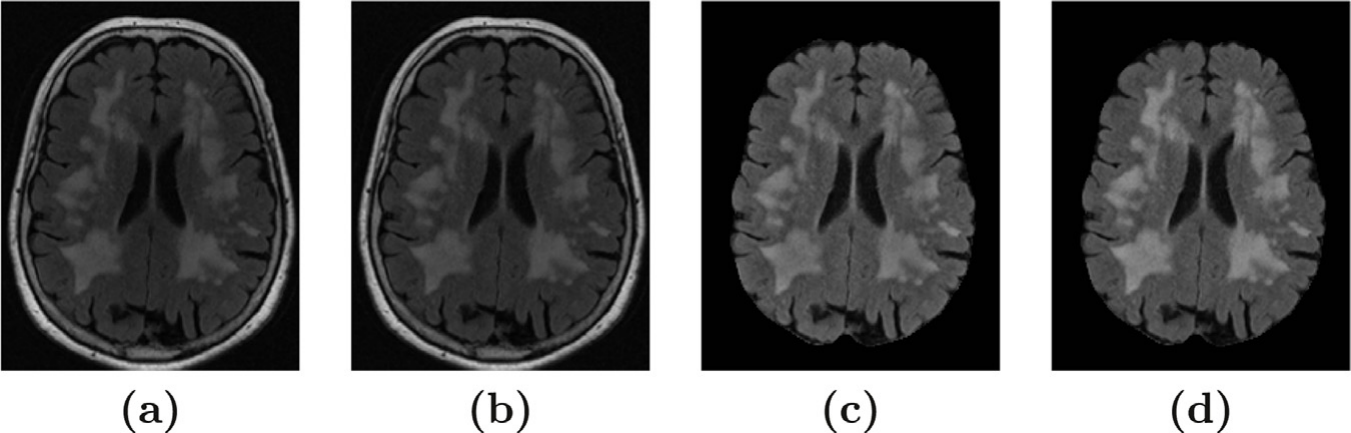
The figure shows the effect of N4 bias correction on a FLAIR image with a large lesion load. (a) The original FLAIR image before skullstripping; (b) after N4 bias correction (with skull); (c) After N4 bias correction (without skull); and (d) After skull-stripping and bias correction using pure-tissue probability mask.
